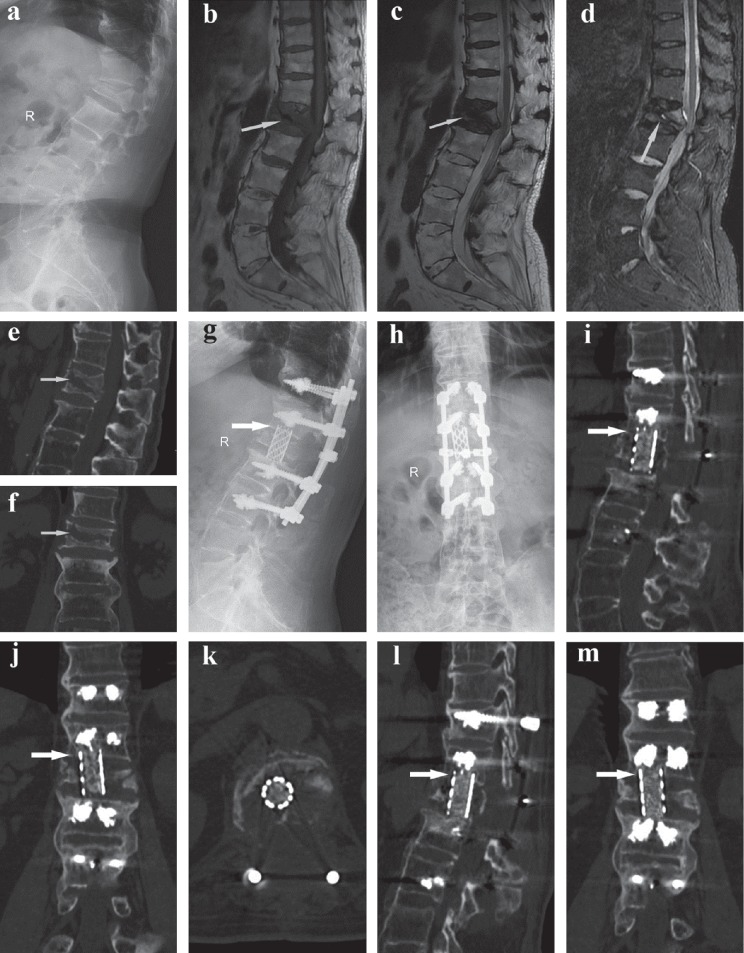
A 67-year-old male patient experienced OVCFs with IVC complicated by neurological deficits and underwent PVCR treatment. (a) Preoperative sagittal plain radiographs revealed collapsed fracture at L1 and kyphosis. (b, d) Sagittal T1- and T2-weighted MRI and STIR MRI scans showed a decreased signal in the intravertebral cleft in L1. (e, f) Coronal and sagittal CT showed an IVC in L1. (g, h) Immediate postoperative plain radiographs showed corpectomy, screw fusion, increased anterior vertebral height, and restored kyphosis. (i–m) Coronal and sagittal CT showed no reduction in vertebral height and no kyphosis recurrence at the 1- and 2-year follow-up examinations.
